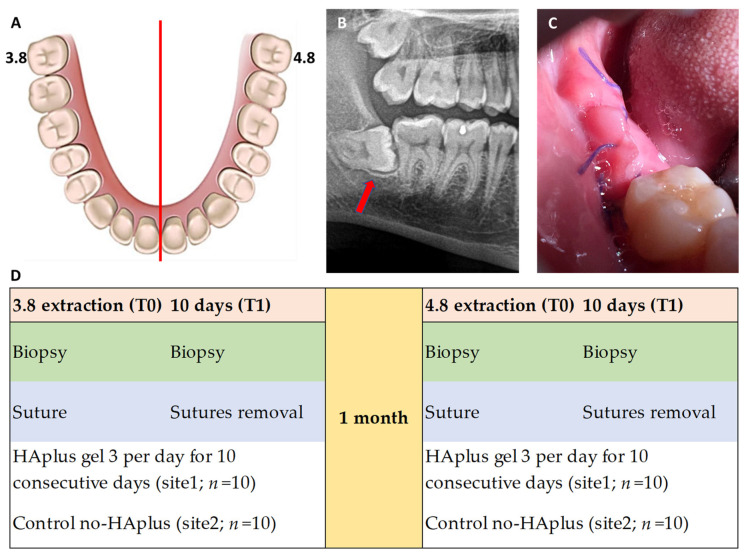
(A) Graphical representation of split-mouth design involving third molars 3.8 and 4.8; (B) red arrow indicates a mesioangular third molar at orthopantomography; (C) intraoral photograph shows post-extractive site at 10 days with flap closure via the Donati suture technique with consequent excesses of tissue to maintain tensile strength and create a stable gingival coverage for better healing of the alveolar socket. (D) Post-extraction treatment regimens tested: test sites were treated with HAplus (Aminogam gel^®^ Errekappa Euroterapici spa) gel, while control sites were not treated with any topical substances (no-HAplus).
